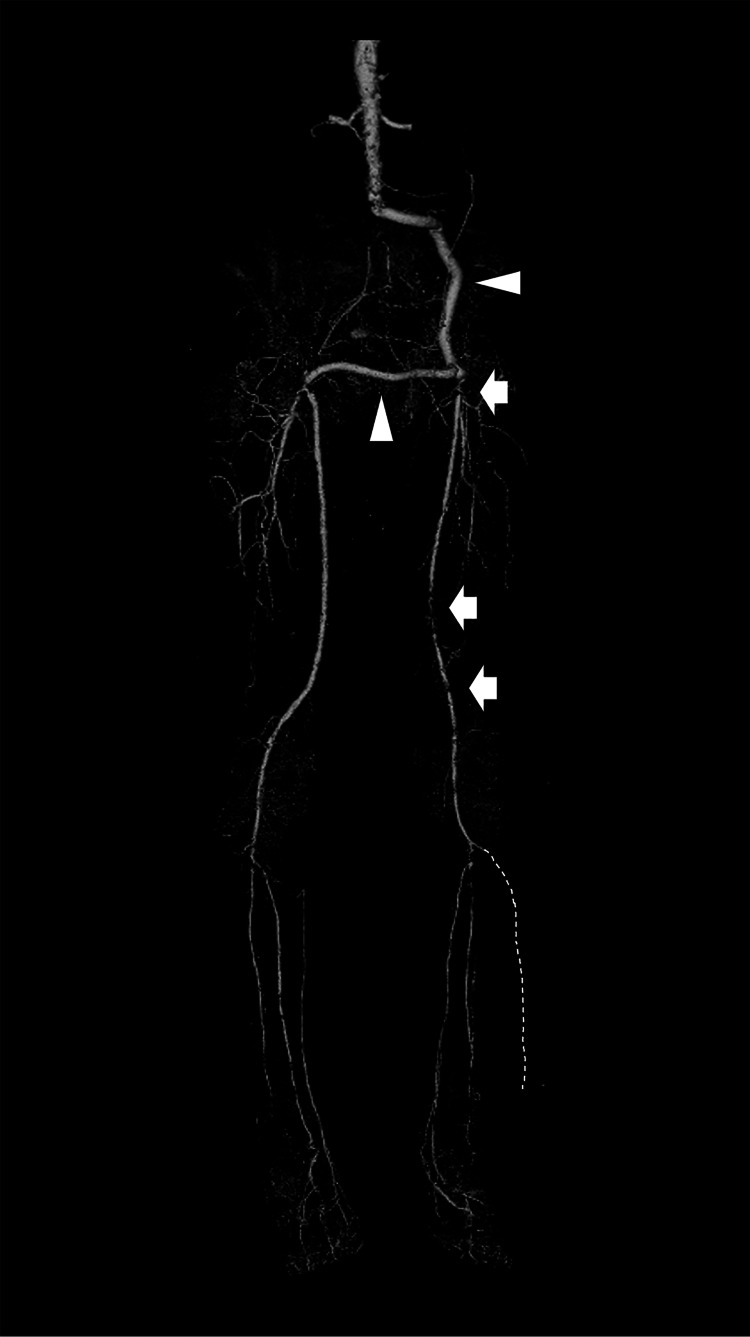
Three-dimensional constructed computed tomography angiography The white arrowheads indicate the prosthesis bypass grafts (femorofemoral and left iliofemoral) without stenotic lesions. The white arrows indicate the stenotic lesions in the left common femoral artery and in the middle and distal parts of the left superficial femoral artery. The left anterior tibial artery was completely occluded in the proximal part as indicated by the white dotted line.
